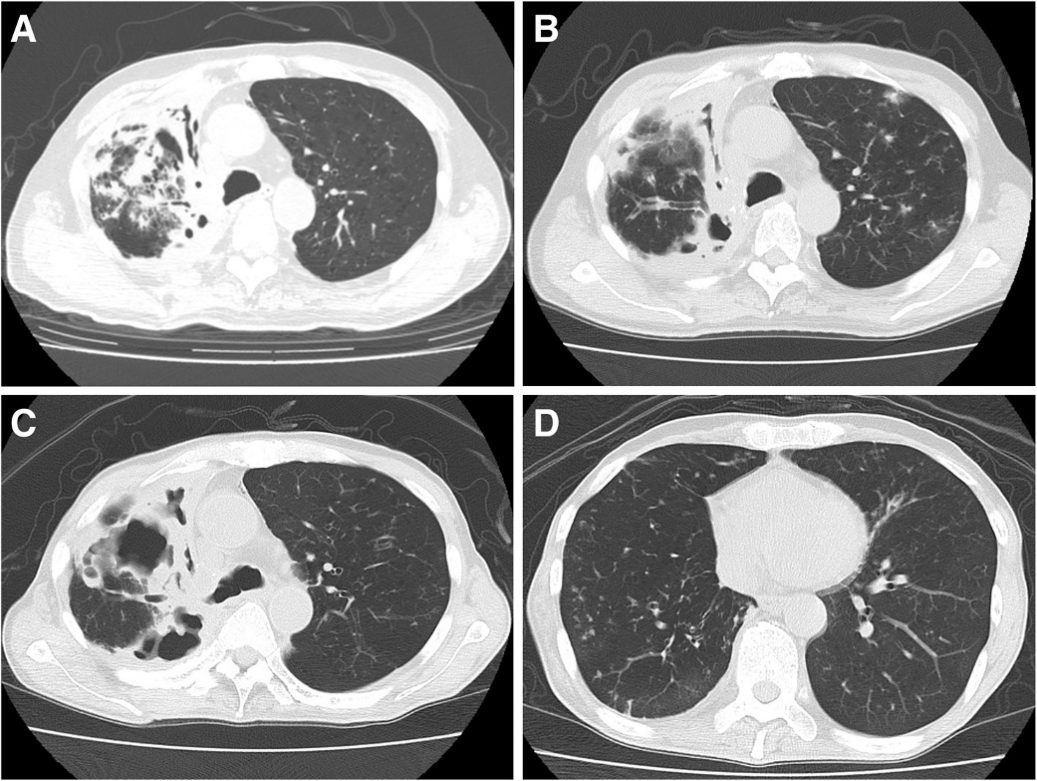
Chest CT course before final admission. (A) On first admission for the treatment of *M. intracellulare* pulmonary infection, showing a infiltrative shadow in the right upper lung field. (B) 1.5 years after *M. abscessus* was first detected in his sputum, revealing bronchiectasis and cavitary lesions in the middle lobe. (C, D) Six months before his final admission, showing bronchiectasis and cavitary lesions progressed towards the bronchial stump (C) and mild lesions in the right lower lobe (D).
